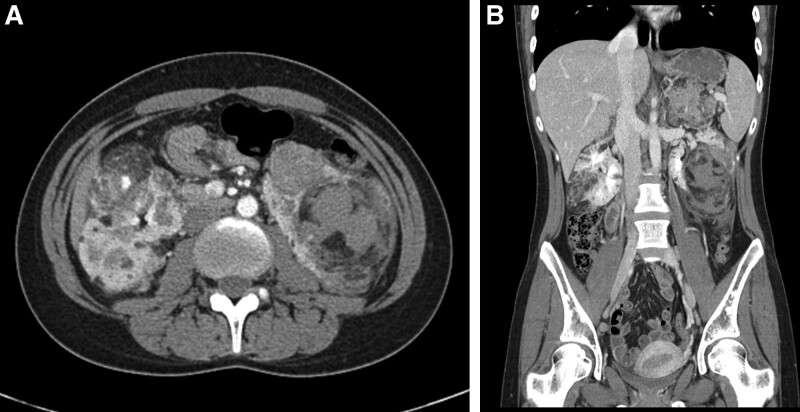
Abdominal computed tomography scan. (A) Axial CT image showing bilateral renal AMLs rupture with an intra-hemorrhagic component. (B) Coronal CT scan demonstrating AML rupture in the left kidney. CT = computed tomography, AML = angiomyolipoma.
